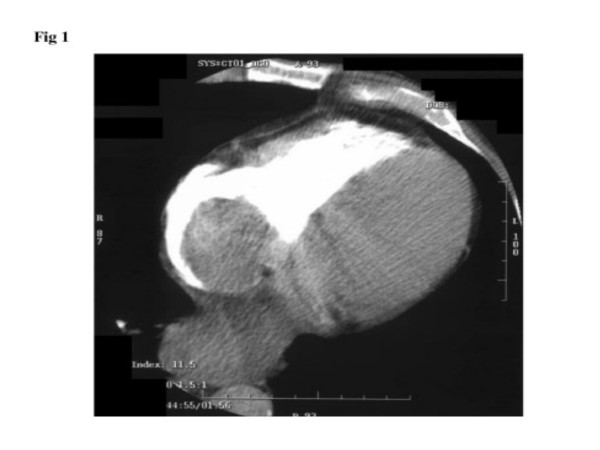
CT scan showing soft tissue mass within atrium which is isodense with myocardium and appearing to arise from the atrial wall by a stalk.
